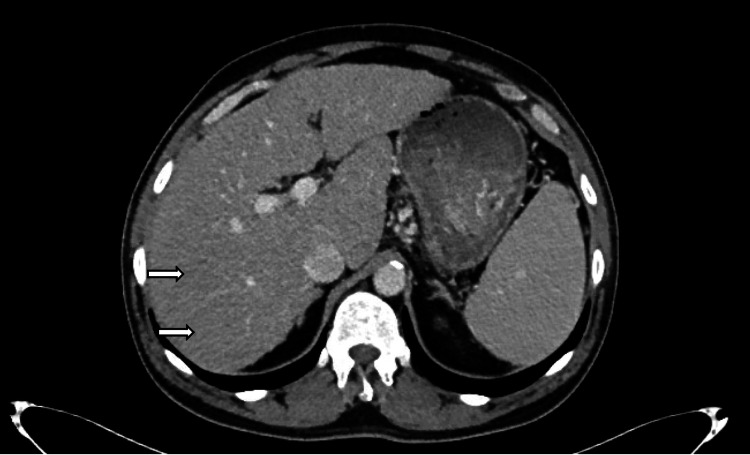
Follow-up contrast-enhanced CT of the abdomen and pelvis showing significant interval improvement in previously noted hepatic lesions. The liver demonstrates a cirrhotic morphology with no new or enlarging lesions. The ring-enhancing lesions have resolved, suggestive of a favorable response to antimicrobial therapy.
